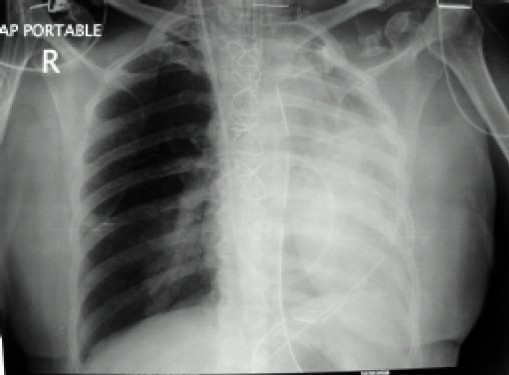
Chest radiograph showing homogenous opacity in left lung field with mediastinal shift towards the left side suggesting left-sided lung collapse.
